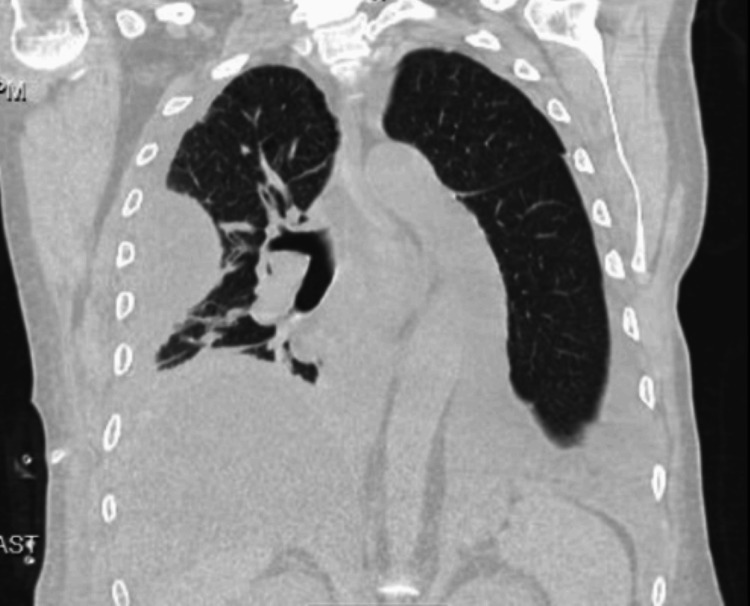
CT chest. Coronal view (Contd.) Showing improved but persistent moderate loculated right pleural fluid with tiny foci of air with a chest drain within the inferior loculation
